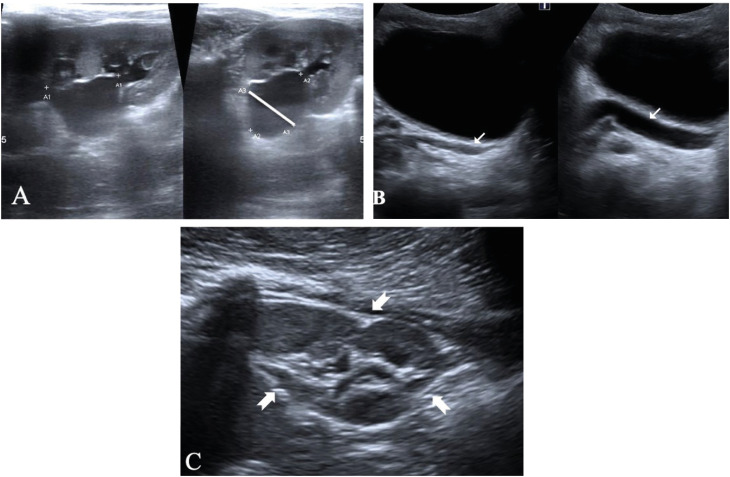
Sonography indexes. (A- renal pelvic diameter, B- distal ureteral diameter, C-renal scarring).
